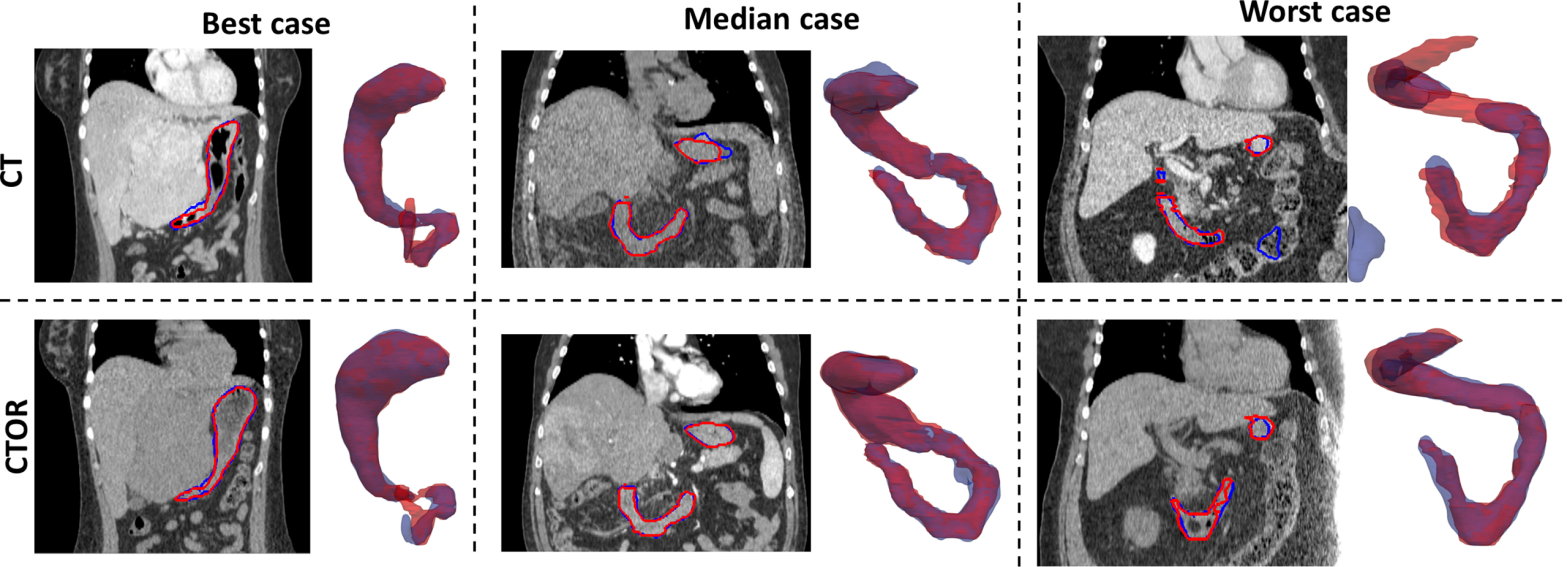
Representation for 3 test cases of manually-drawn (in red) and auto-segmented (in blue) contours of the stomach and duodenum combination on transversal slices of the images and 3D perspective (posterior to anterior direction). Top row: CT scans; Bottom row: CTOR scans.
